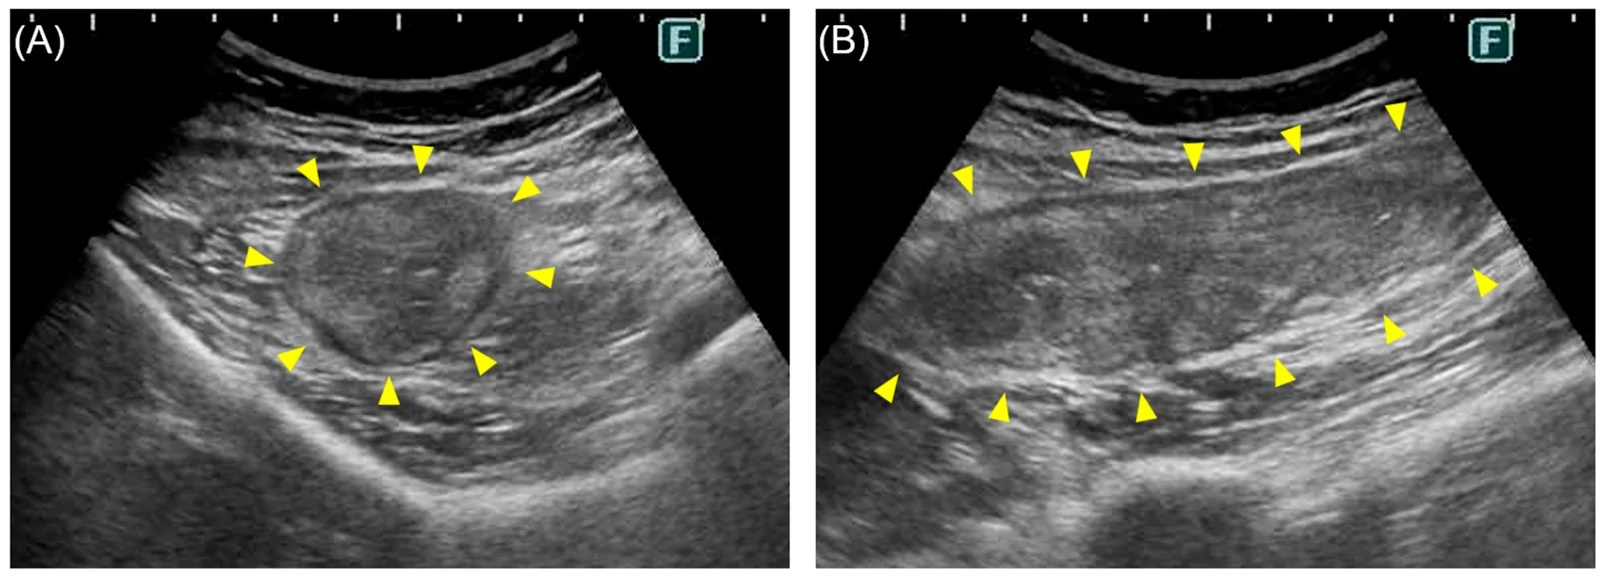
Abdominal ultrasonography on admission. (A) Short-axis and (B) long-axis views of the ascending colon showing marked circumferential bowel-wall thickening of approximately 2 cm (yellow arrowheads) with loss of normal bowel-wall stratification. Similar changes extended continuously from the ascending colon to the proximal transverse colon. This case represents a rare coinfection with EHEC O157 and *Campylobacter jejuni* following raw beef consumption. Confirmed coinfection with these pathogens is extremely rare; for instance, only one case was identified among 775 suspected patients during the 1999 New York waterborne outbreak [1]. In our patient, abdominal ultrasonography played a central diagnostic role by clearly demonstrating severe right-sided colitis and delineating the continuous extent of bowel involvement. Given her young age and clinical stability, abdominal computed tomography (CT) was omitted to avoid unnecessary radiation exposure, as ultrasonography provided sufficient diagnostic detail to guide immediate management. Although CT remains a valuable complementary tool when ultrasonographic visualization is limited by bowel gas or when surgical complications are suspected, neither ultrasonography nor CT can differentiate EHEC O157 from *Campylobacter jejuni*, nor can imaging identify the presence of a coinfection. This rare dual infection created a critical therapeutic challenge. Clinical guidelines consider antimicrobial therapy (e.g., macrolides) for severe *Campylobacter* enteritis presenting with severe abdominal pain and bloody stools; however, antimicrobial use in EHEC O157 infection is strictly discouraged due to the potential risk of triggering hemolytic uremic syndrome (HUS) [2]. Consequently, the identification of EHEC O157 dictated a conservative strategy, precluding targeted antibiotic treatment for *Campylobacter*. Although some studies in pediatric populations suggest that polymicrobial enteric infections may be associated with increased disease severity [3], our patient—a previously healthy young adult—achieved full recovery with supportive care and probiotics alone. This case highlights ultrasonography as an effective, non-invasive primary imaging modality for assessing the extent of acute right-sided colitis, and demonstrates that cautious supportive management can achieve favorable outcomes even when conflicting guidelines constrain antimicrobial choices.
